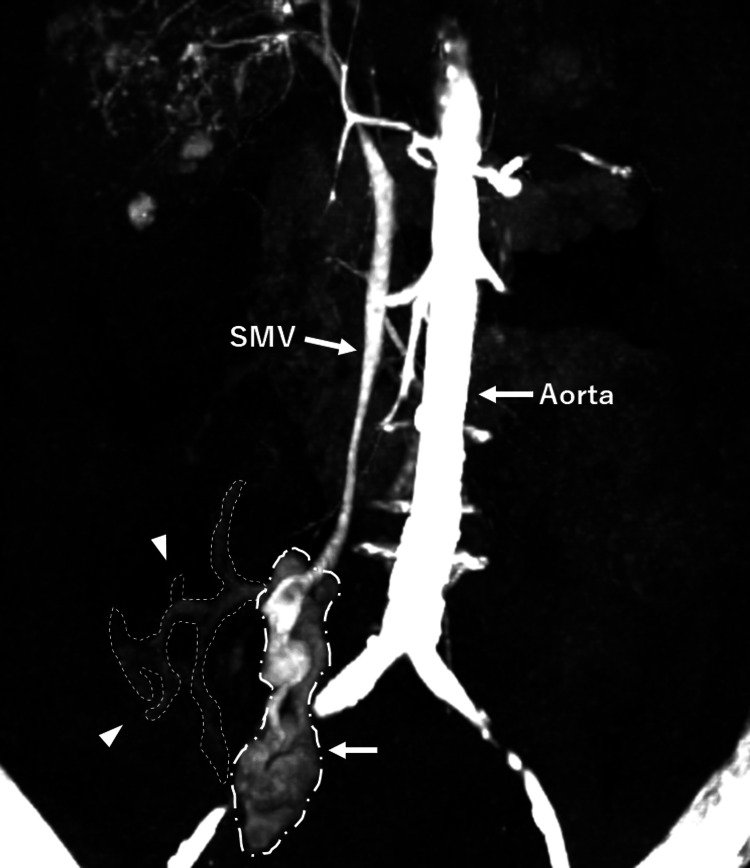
Three-dimensional reconstruction of abdominal CT scan showing rupture of the ectopic superior mesenteric vein varix This reconstructed CT image clearly reveals the superior mesenteric vein, the ruptured superior mesenteric vein (SMV) varices (arrows), and the associated extravasation and intraperitoneal hemorrhage (arrowheads).
